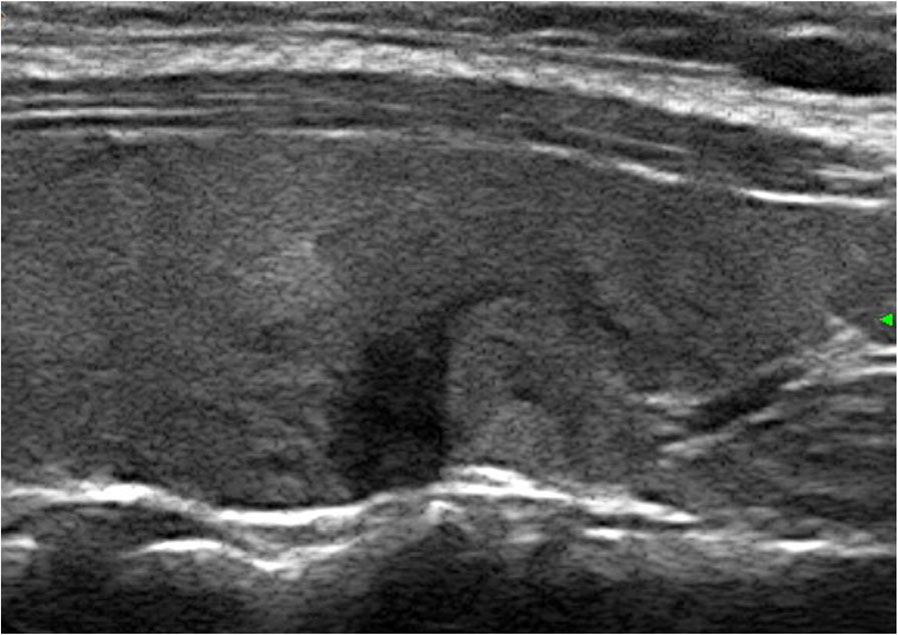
A 40-year-old female with PTMC. The longitudinal sonogram of the tumor presented a taller than wide shape and speculated margin
